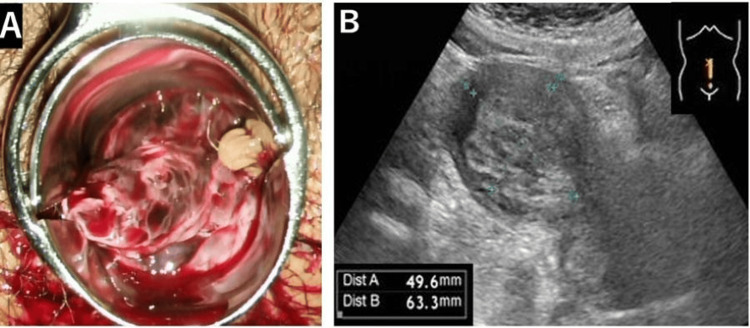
Findings on clinical examination. A) Speculum examination: a fragile, hemorrhagic mass containing small cystic components was observed prolapsing beyond the external cervical os into the vagina. B) Transabdominal ultrasound findings: a 50×63 mm mass containing small cystic components was observed within the uterine cavity.
